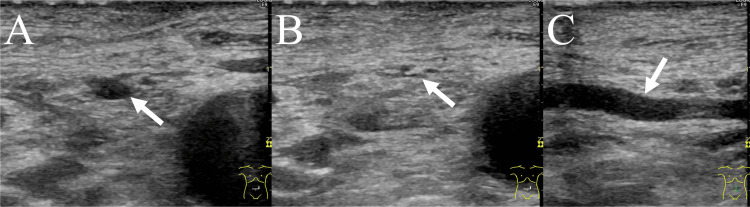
Ultrasound images of the jejunal vein continuous with the jejunal varices at the hepaticojejunostomy site (A) The ultrasound image in the transverse plane clearly visualizes the jejunal vein (arrow) without overlying bowels. (B) The jejunal vein (arrow) becomes flattened upon gentle compression. (C) Ultrasound image in the sagittal plane reveals that the jejunal vein (arrow) runs straight, and a puncture of the vein seems feasible.
